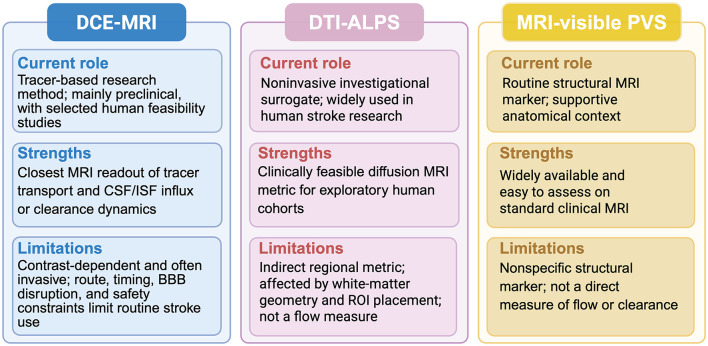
Comparison of three MRI readout classes used in post-stroke glymphatic and meningeal lymphatic research. DCE-MRI provides tracer-based information on CSF/ISF transport but is usually contrast-dependent, often invasive, and mainly supported by preclinical or selected feasibility studies. DTI-ALPS is a noninvasive diffusion MRI surrogate widely used in human stroke research, but it does not directly measure bulk flow or clearance. MRI-visible PVS provide routine structural context but are nonspecific and should not be interpreted as standalone evidence of glymphatic dysfunction. DCE-MRI, dynamic contrast-enhanced magnetic resonance imaging; DTI-ALPS, diffusion tensor image analysis along the perivascular space; PVS, perivascular spaces; CSF, cerebrospinal fluid; ISF, interstitial fluid. Created in BioRender. Jin, Y. (2026) https://BioRender.com/k4kqr3j.
